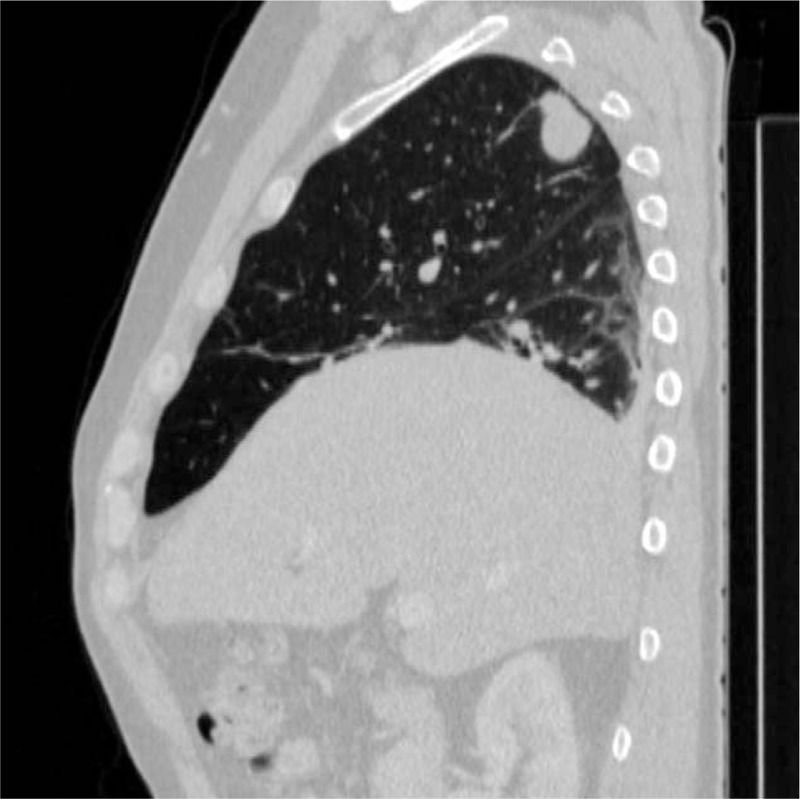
CT scan of the thorax showing the presence of a mass lesion located in the dorsal segment of the right upper pulmonary lobe – sagittal sections. CT = computed tomography.
